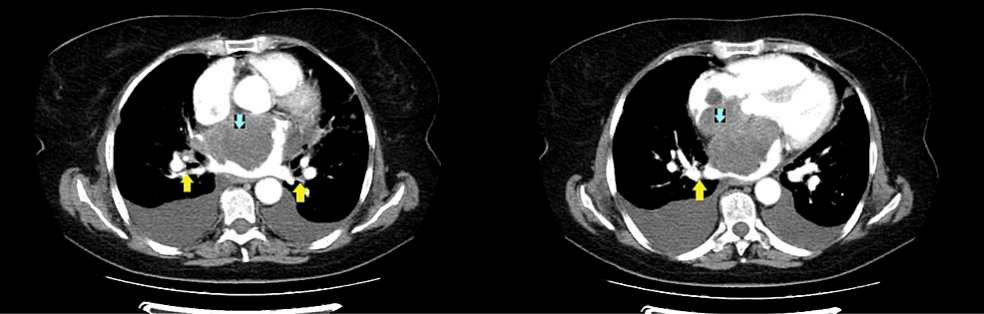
Thorax CT scan with contrast (transverse section) showing a tumor in the left atrium (blue arrows). The tumor is compressing the pulmonary veins (yellow arrows).
